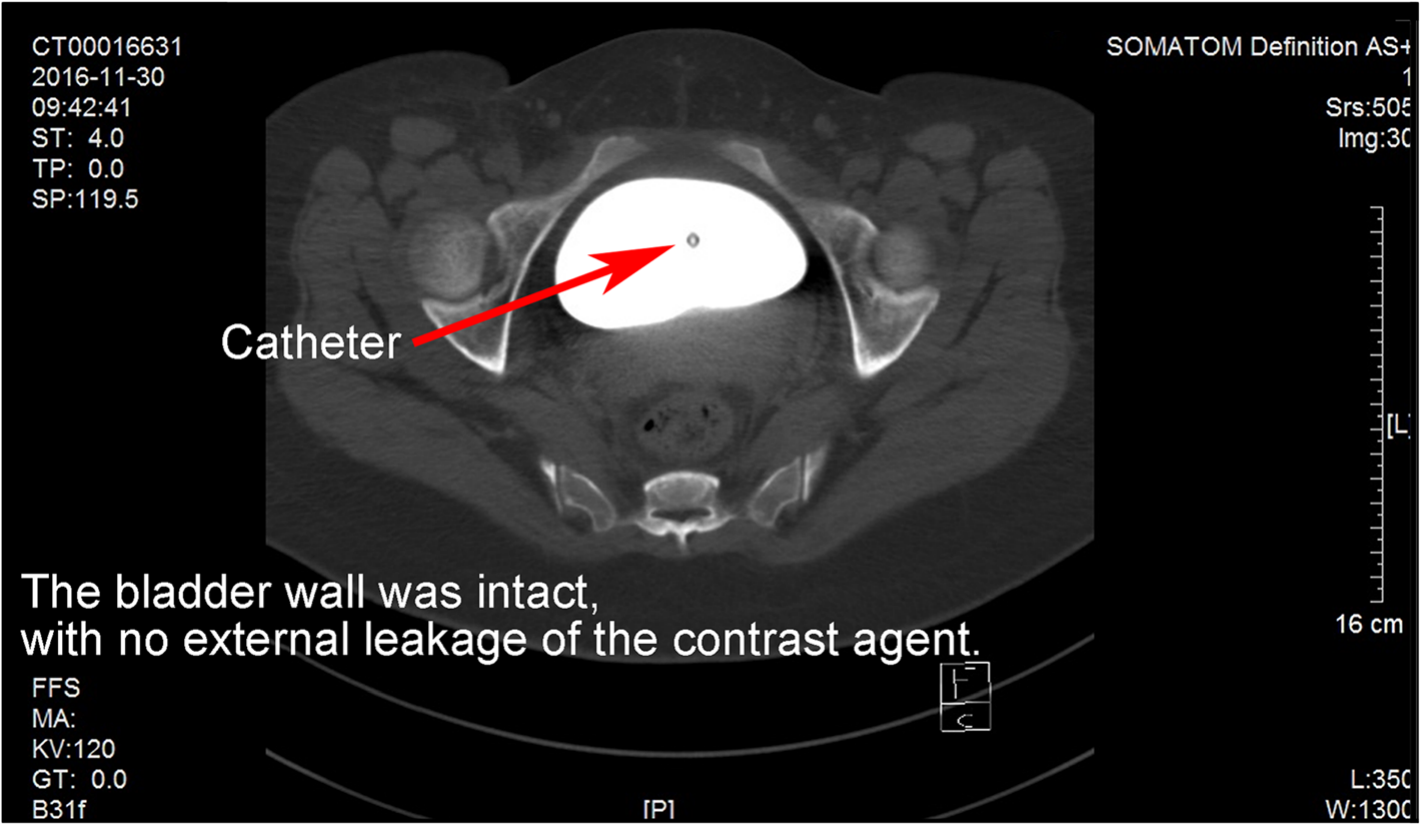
CT bladder radiography. 250 mL of contrast agent was injected via a catheter. The image shows no extravasation of the contrast agent
